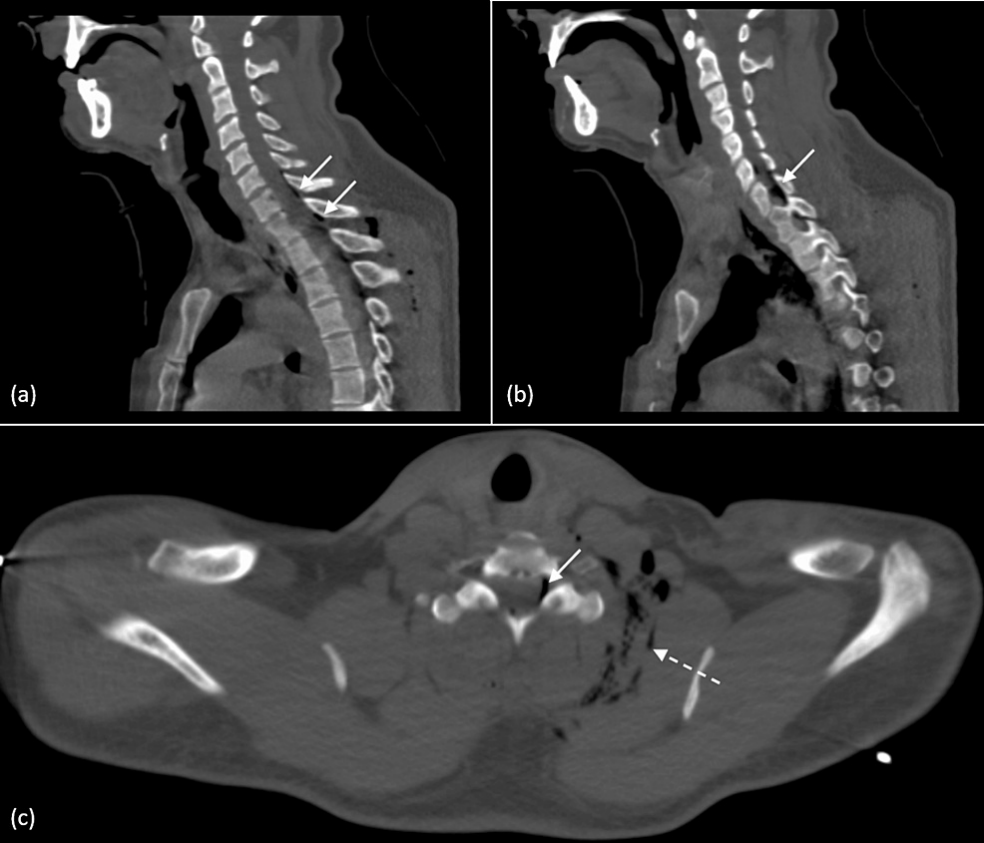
(a-b) Non-contrast thoracic CT sagittal images in bone window setting, displaying epidural pneumorrhachis (solid white arrow). (c) Axial cut in bone window setting, displaying left cervical subcutaneous emphysema (dashed white arrow) and epidural pneumorrhachis (solid white arrow). CT: computed tomography
